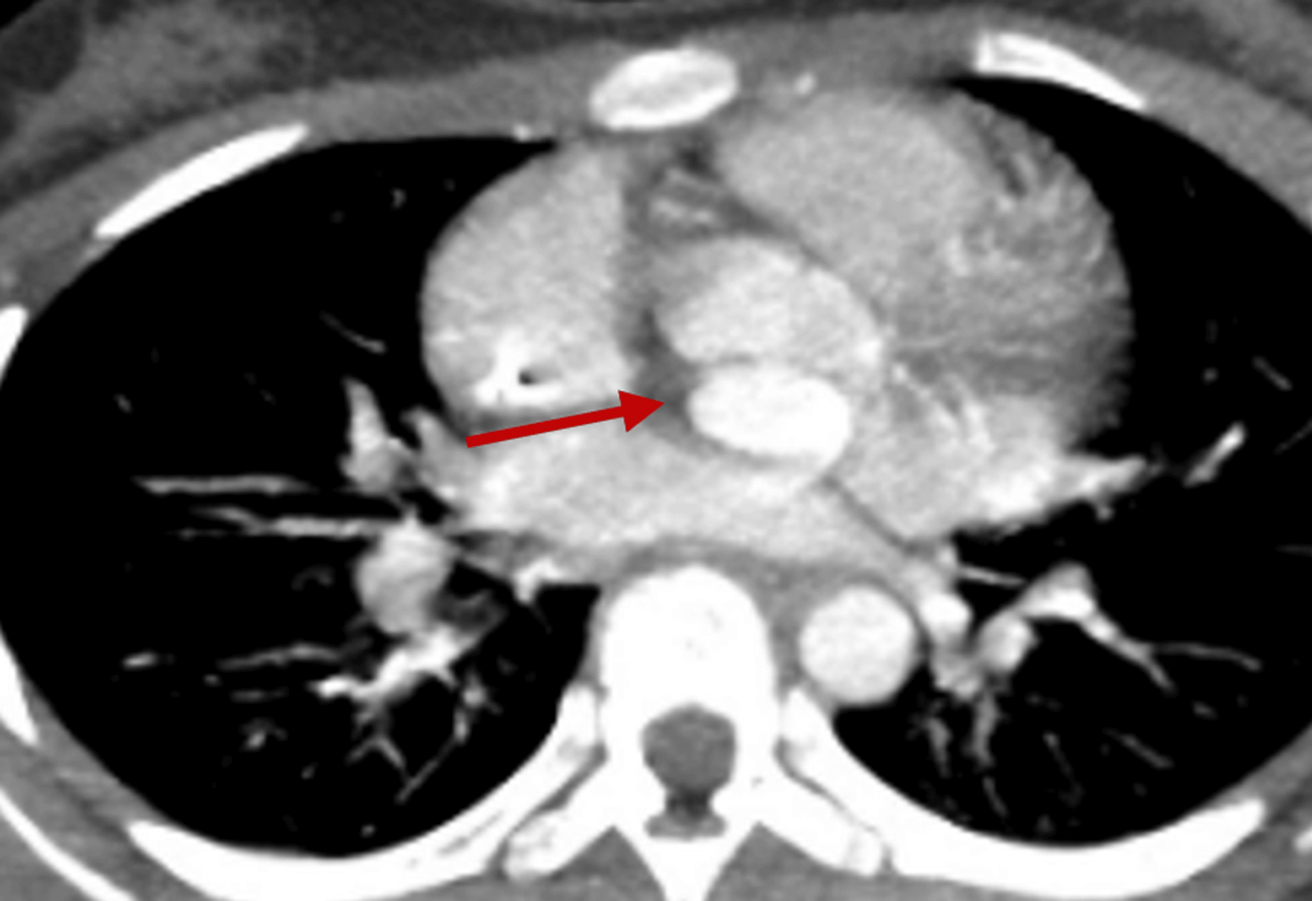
Chest CT (axial view) demonstrating the same lesion described in Figure 6, indicated by the red arrow, highlighting its spatial relationship with surrounding cardiac structures
